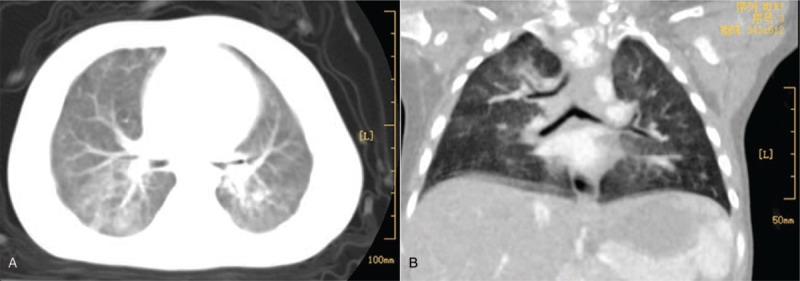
Chest CT with computed tomography angiography of the 10-month-old boy with the VACTERL association. The CT (A) and computed tomography angiography (B) indicated bridging bronchus and tracheal stenosis in the left main bronchus.
